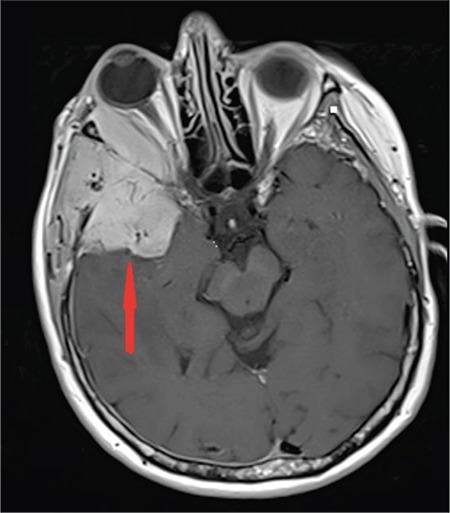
Cranial axial contrast magnetic resonance image before treatment: in the lateral aspect of the right orbit there is a mass lesion that expands and destroys the zygomatic bone and temporal lobe (red arrow). The mass lengthened in the cerebral parenchyma by invading the dura in the temporal region.
